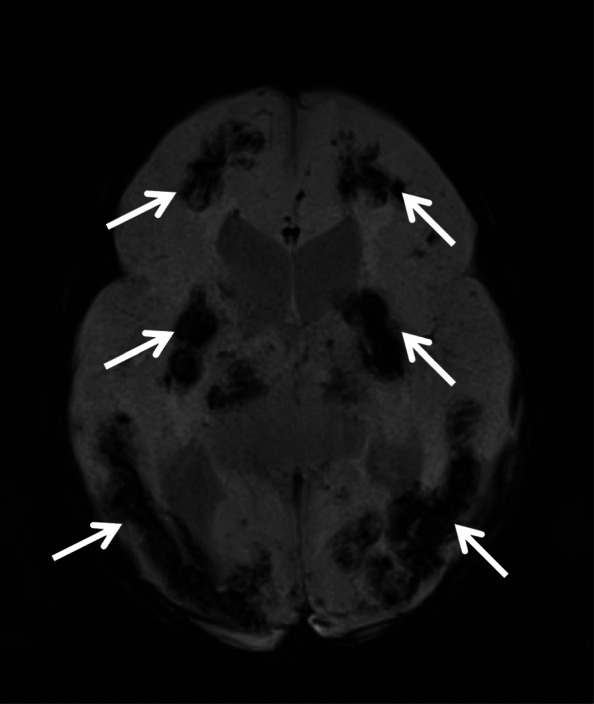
Diffuse symmetric calcifications in MRI
